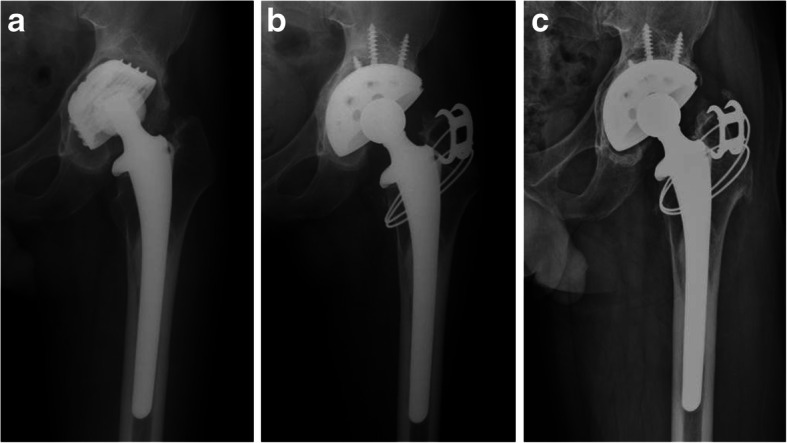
(a) Preoperative radiograph showing aseptic loosening of acetabular cup; (b) Postoperative radiograph of cementless total hip arthroplasty performed with Longevity higly cross-linked polyethylene liner and 28-mm metal head; (c) Twelve-year follow-up radiograph showing well-fixed prostheses without osteolysis. The polyethylene liner wear rate was 0.014 mm/year
